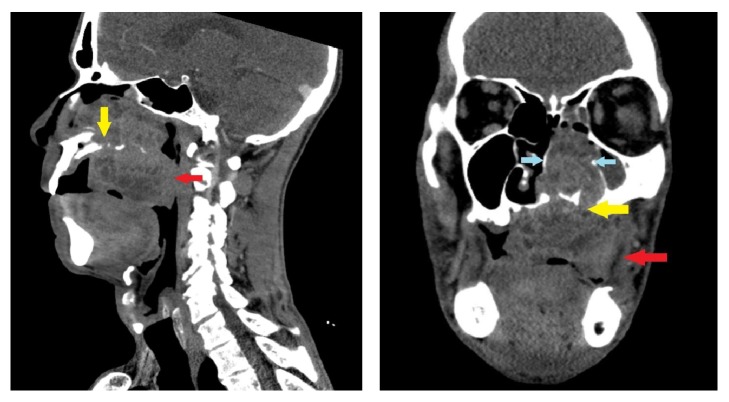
Sagittal and coronal contrast-enhanced CT images of the neck show a large, heterogeneously enhancing and locally aggressive mass centered at the hard palate to the left of midline. Mass erodes the hard palate (yellow arrow) and extends superiorly into the nasal cavity with extensive bony remodeling (blue arrows). Large exophytic component projects into the oral cavity (red arrow).
